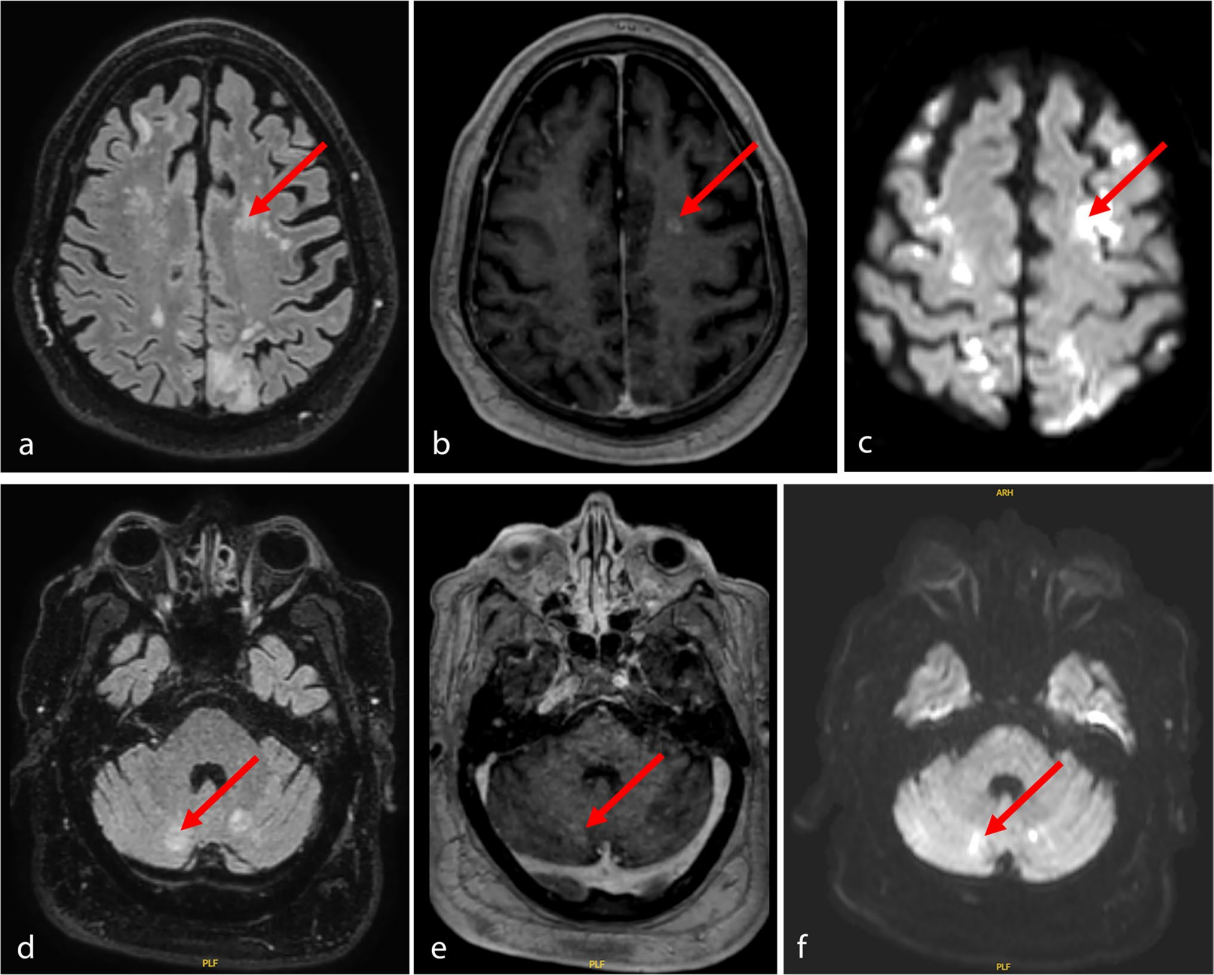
Axial cranial MRI one day after petroleum exposition. Imaging revealed both supratentorial (upper row) and cerebellar (lower row) FLAIR hyperintense (a and d), T1-Gadolineum-enhancing (b and e) diffusion-restricted (c and f) lesions, suspicious for cytotoxic edema (red arrows, each). In contrast, contrast-enhancing lacunar infarctions were considered unlikely due to the short duration between exposition/onset of the symptoms and imaging
